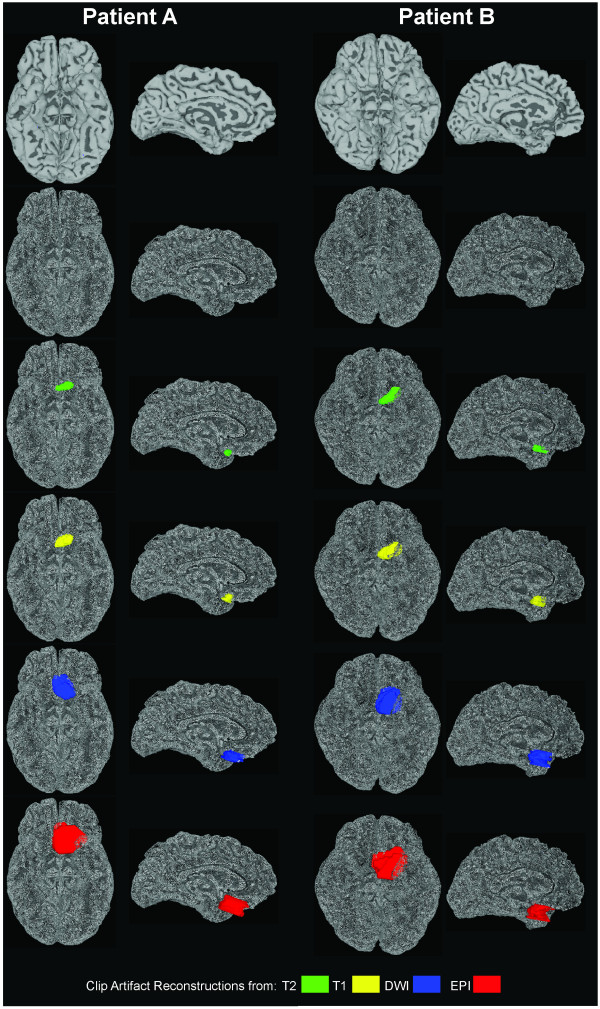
Example Clip Artifact Volume Reconstructions in Relation to Cortical Surface Models. Basal and mid-sagittal views of each patient's cortical surface model are shown shaded by curvature (top row) and as a point cloud (second row). Superimposed on the point could displays to aide artifact visualization are the reconstructed clip artifact volumes colored according to MR sequence in descending order by clip volume as percentage of brain volume (T2 = green, T1 = yellow, DWI = blue, EPI = red). These artifact volumes are 0.27%, 0.56%, 1.70%, and 4.67% respectively for Patient A (two columns on left), and 0.48%, 1.04%, 3.62%, and 4.74% respectively for Patient B (two columns on right).
